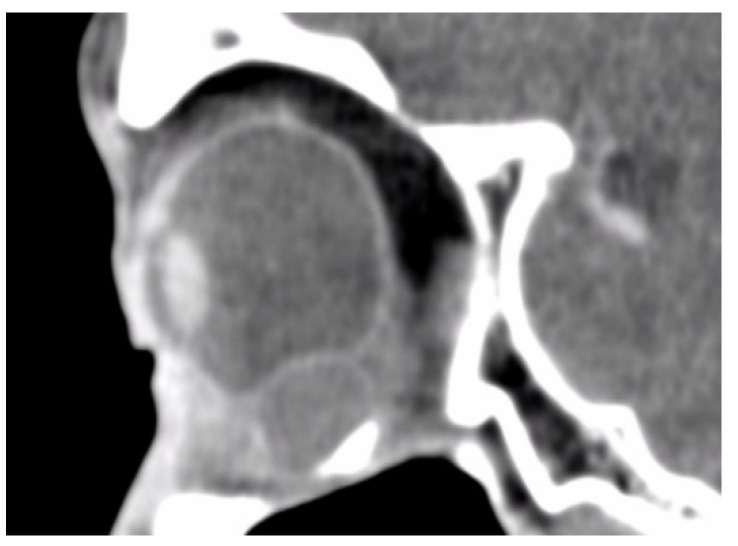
Orbital CT at sagittal view revealed posteriorly located hydrogel scleral buckles.
